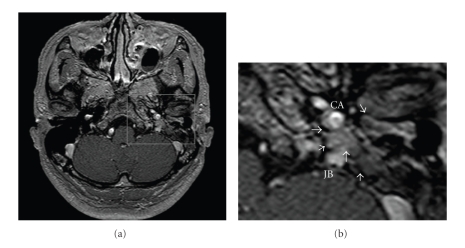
Dynamic enhanced MRI. The mass close to the carotid artery and jugular bulb through the tympanum and mastoid was enhanced in the early phase of the dynamic MRI (white arrows).
